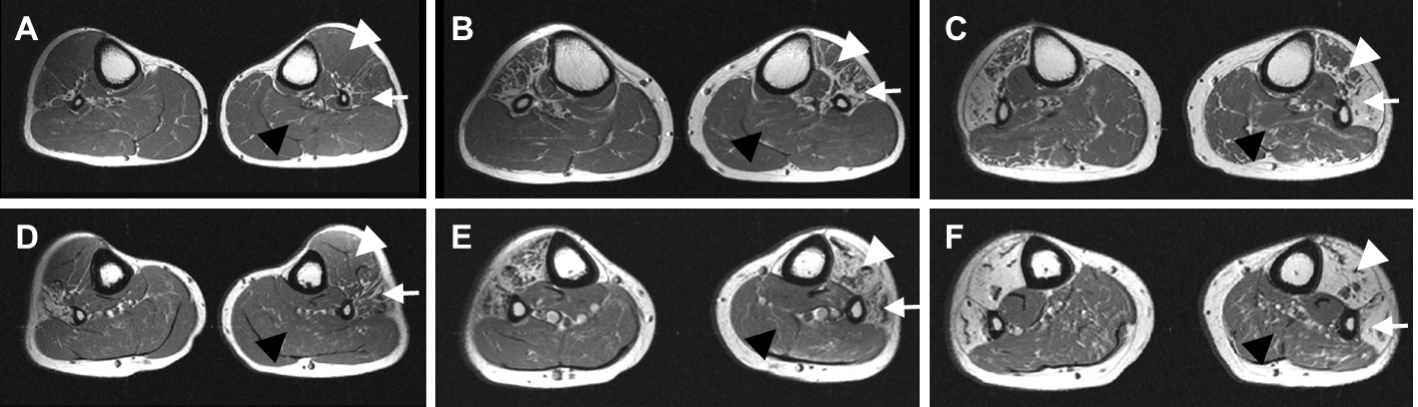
T1-weighted axial MRIs of the upper third (A-C) and lower third (D-F) calf in three patients. (A, D) III-3 patient at the age of 30 years with disease duration (DD) of 22 years. (B, E) III-1 patient at the age of 32 years with DD of 26 years. (C, F) II-4 patient at the age of 56 years with DD of 38 years. A sequential pattern of muscle involvement associated with disease duration was observed. In the early disease stage, the lateral compartment muscles (arrow) including the peroneus longus and brevis were initially involved, and they showed the most severe fatty hyperintense signal changes. A lesser degree of fatty involvement was observed in the anterior compartment muscles (white arrowhead) including the tibialis anterior, extensor hallucis longus, and extensor digitorum longus. In later stages, the posterior compartment muscles (black arrowhead) including the soleus, gastrocnemius, and tibialis posterior in the calf were relatively unaffected.
